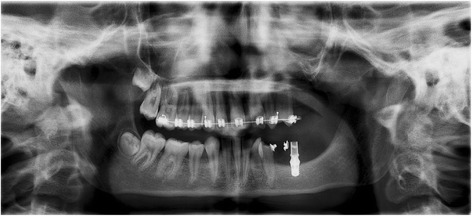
Patient 2—post-operative orthopantomogram (OPT) at age of 13. Situation 10 months after implant placement. Three months after starting the orthodontic treatment, the 34 is already erected
